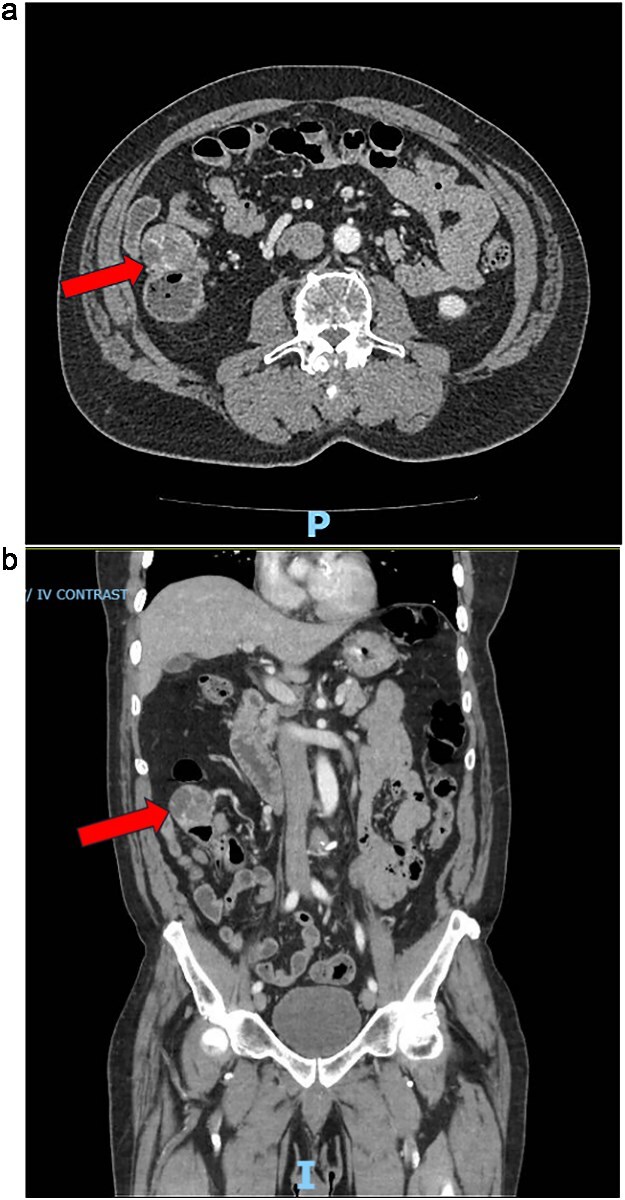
(a) CT abdomen/pelvis axial and coronal views during emergency department presentation with cecal mass seen. (b) CT abdomen/pelvis axial and coronal views during emergency department presentation with cecal mass seen.
